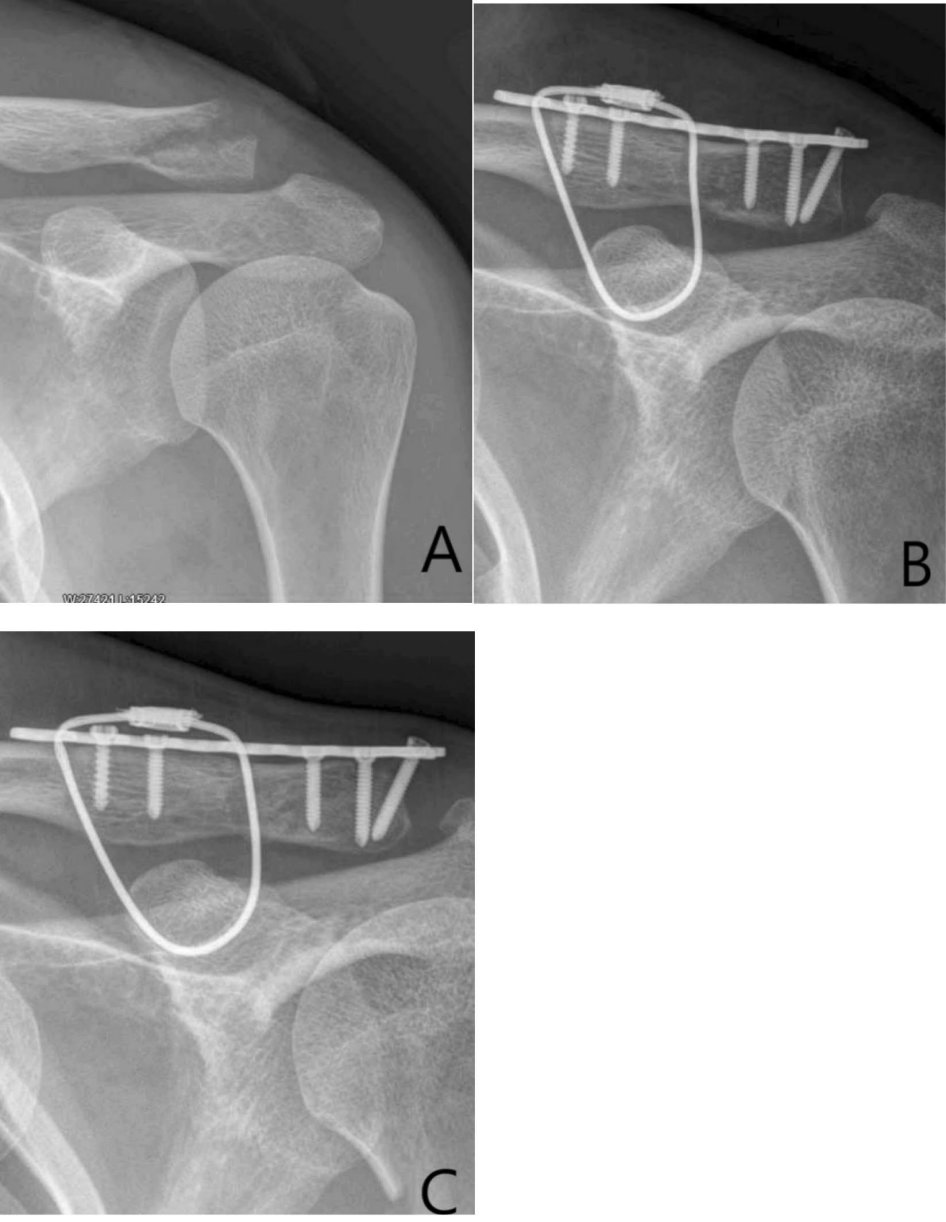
(A–C) A 36 years old woman with left distal clavicle fracture. The distal fragment less than 2.5 cm, a titanium cable was inserted and the metacarpal locking plate was fix. (A) Preoperative X-ray of the shoulder; (B) postoperative X-ray, (C) 3 months after surgery show fracture union.
